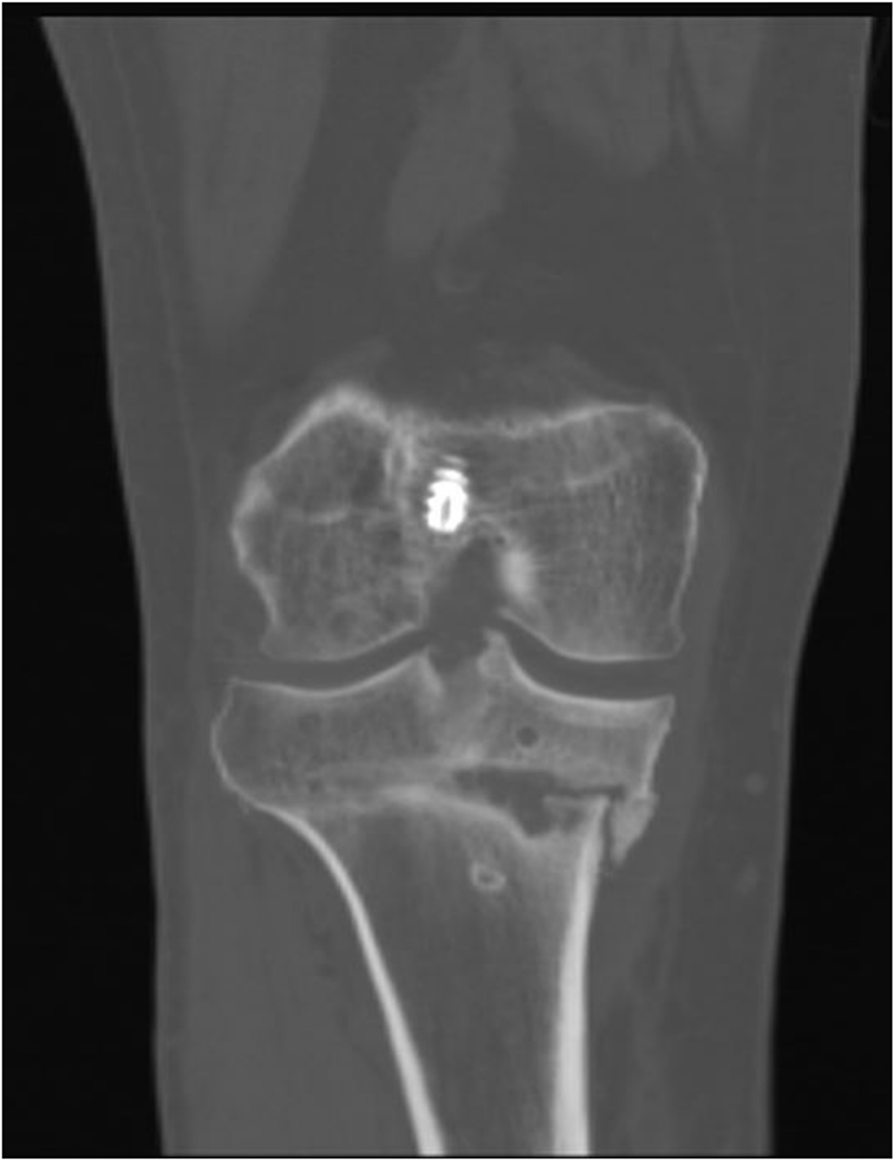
A coronal CT slice demonstrating varus collapse of the osteotomy with nonunion
